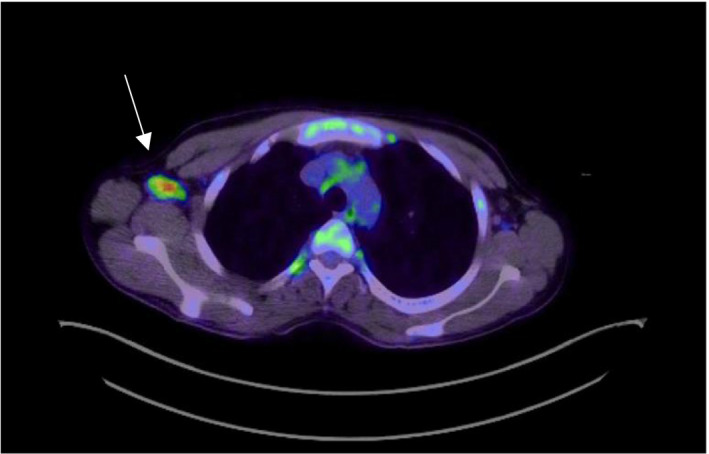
Hypermetabolic right axillary lymph node, 1 cm size, SUV up to 6.9. (White arrow)
